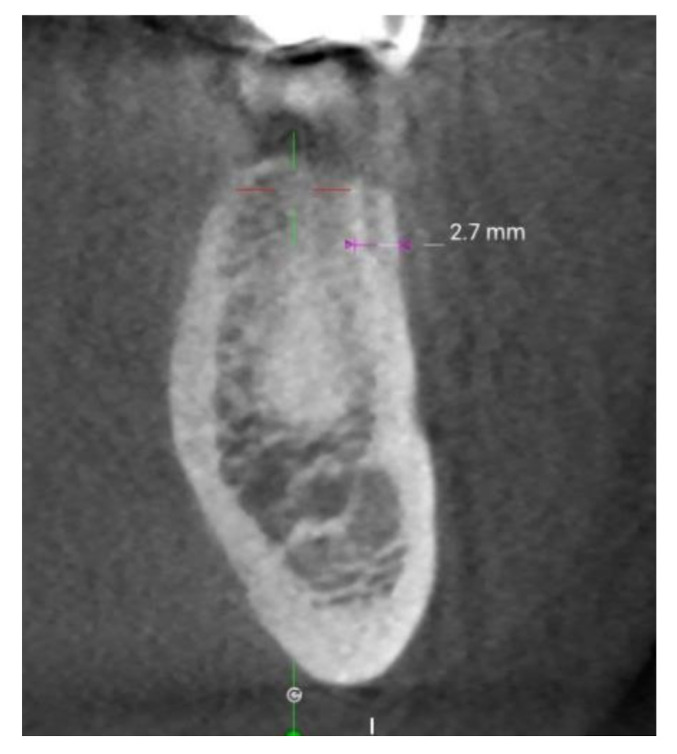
Cortical bone thickness can be easily measured on CBCT scans. The picture shows an axial section of a lower jaw. By using a dedicated software, the surgeon draws a measuring line across the cortical bone at the site to be measured; the software—in its measurement mode—provides the thickness reading directly on the screen.
